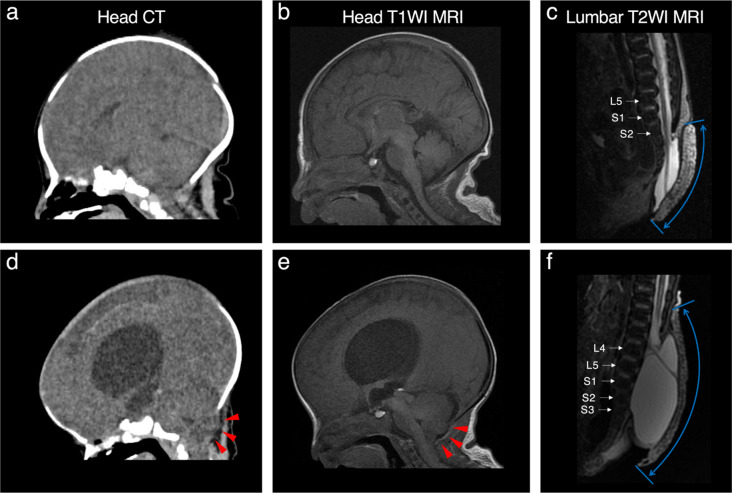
Representative CT and MRI images from non-CM-II or CM-II infants. For a non-CM-II infant, sagittal midline views of head CT (obtained on day 0 after birth) (a), head T1WI MRI (day 4) (b), and lumbar T2WI MRI (day 0) (c) are shown. No cerebellar displacement is observed. The MMC lesion extends from S3 to S5, classified as a sacral type. For a CM-II infant, sagittal midline views of CT (day 0) (d), head T1WI MRI (day 7) (e), and lumbar T2WI MRI (day 0) (f) are presented. Cerebellar displacement through the foramen magnum is observed on both head CT and MRI (indicated by red arrowheads). The MMC lesion extends from L4 to S3, classified as a lower lumbar type. The areas indicated by blue arrows in the lumbar T2WI MRI images (c and f) show gauzes protecting the MMC lesions. CM-II, Chiari malformation type II; CT, computed tomography; MMC, myelomeningocele; MRI, magnetic resonance imaging; T1WI, T1-weighted image; T2WI, T2-weighted image
